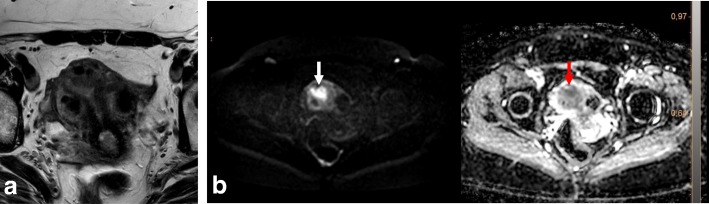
Uterus of postmenopausal woman exhibits myomas and FIGO IA endometrial cancer. Endometrial tumor is not delineated on axial oblique T2WI images (a) and is well-defined on DWI (*b* = 1000, ADC map) (arrows) (b)
